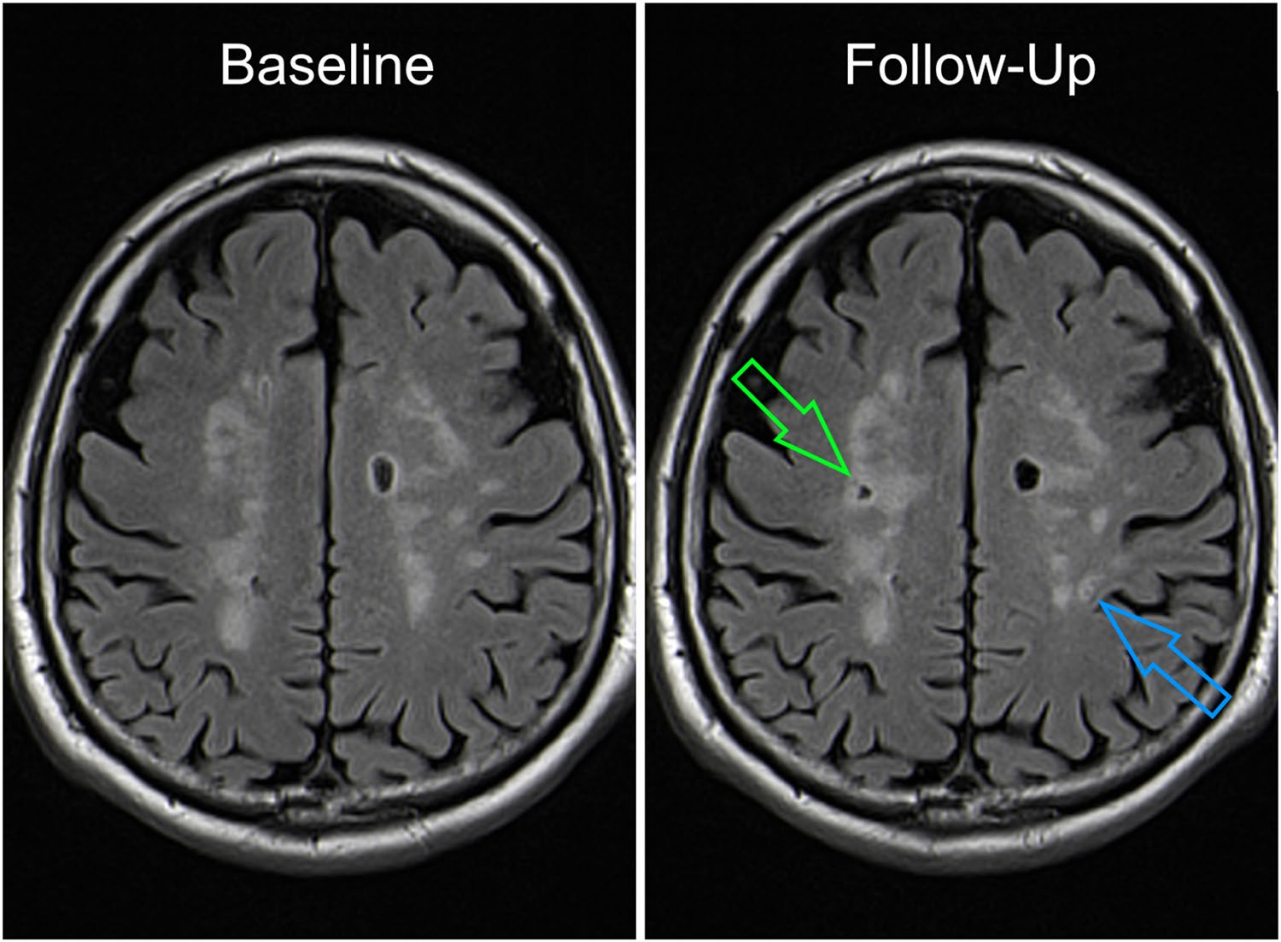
Brain MRI (FLAIR sequence) of a 72-year-old male patient with progression of cerebral small vessel disease during the observation period. The left image shows the baseline MRI (recent infarct not shown), the right image the follow-up MRI after fifteen months depicting a new lacune in the right centrum semiovale (green arrow) and a new white matter hyperintensity in the left centrum semiovale (blue arrow), which occurred clinically asymptomatic. Aside from well-controlled arterial hypertension, this patient had no further vascular risk factors. Baseline eGFR was 68 ml/min/1.73 m², the patient had no increased albuminuria/ACR, but FGF-23 was increased at 159 rU/mL. Follow-up laboratory assessments showed decreasing eGFR (45 ml/min/1.73 m² at fifteen months) and increasing FGF-23 (168 rU/mL at fifteen months).
